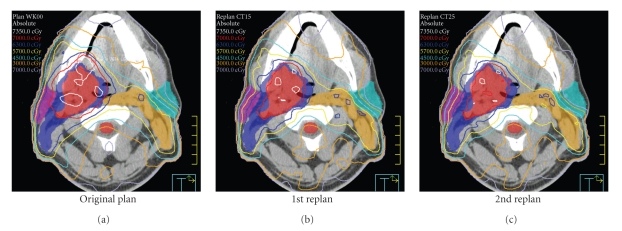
An example of serial ART dose recalculation using a daily CT image acquired at the 25th treatment fraction. On (a), the original plan is calculated on current anatomy. The original plan provides inappropriate treatment margins and dose heterogeneity within the high-dose CTV. In the (b), an earlier ART replan (ART1, designed at the 15th treatment fraction) is calculated onto current anatomy. On (c), a 2nd ART replan (ART2) is designed and calculated for the current daily image set. The ART2 plan provides improved contralateral parotid sparing and a lower total body dose than the ART1 plan.
